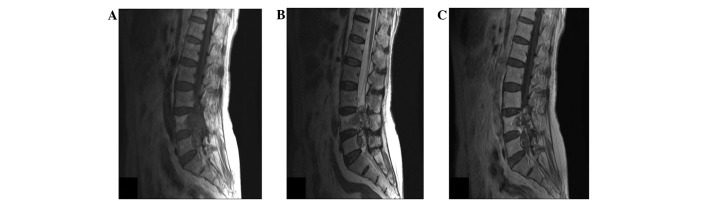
(A) T1-weighted sagittal image showing the hypointense lesion involving the L4 and L5 vertebrae with local bone destruction. (B) T2-weighted sagittal image showing the hyperintense lesion. (C) Post-contrast T1-weighted sagittal image showing the heterogeneous enhancement of the lesion.
